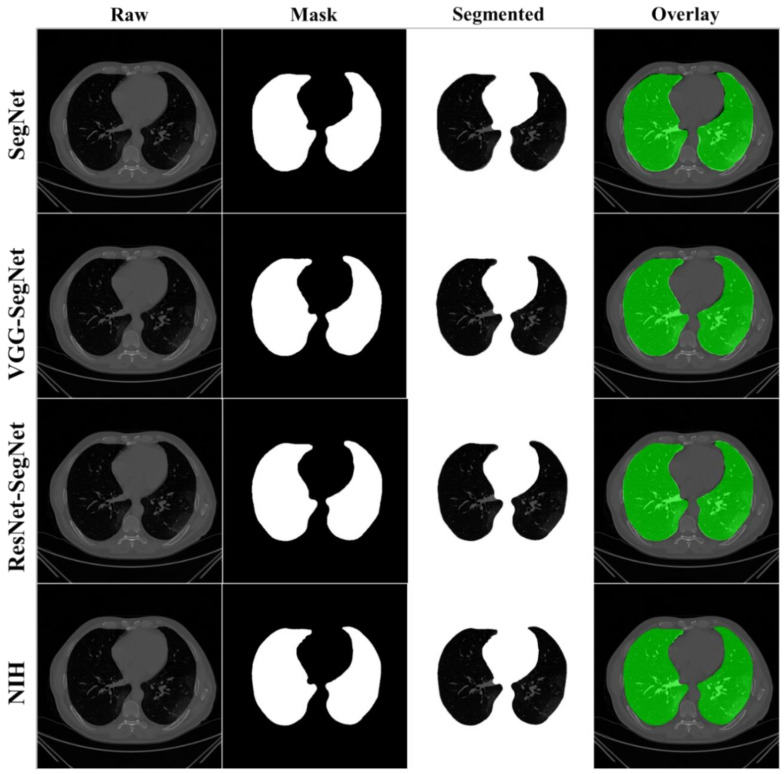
Results from the four AI models with grayscale CT slice. Row 1 to Row 4 is four models: SegNet, VGG-SegNet, ResNet-SegNet, and NIH.
